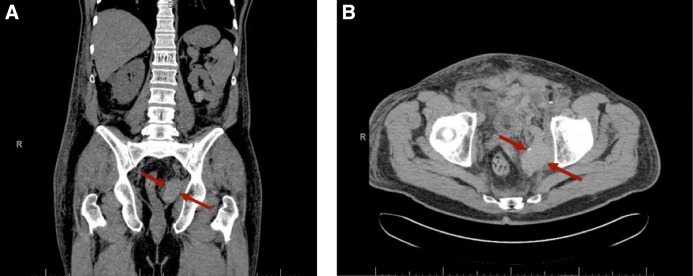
Postoperative CT scans. (A) Coronal section. (B) Axial section. *Red arrows* indicate expansile hematoma in the left paracolic gutter.
